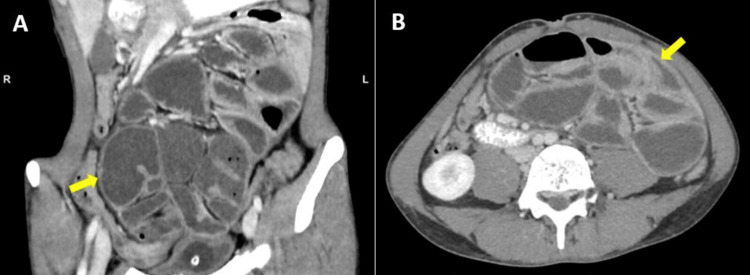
Coronal reconstruction (A) and axial slice (B) of abdominal CT with contrast showing clumped and dilated small bowel loops surrounded by an enhancing membrane (yellow arrows).
